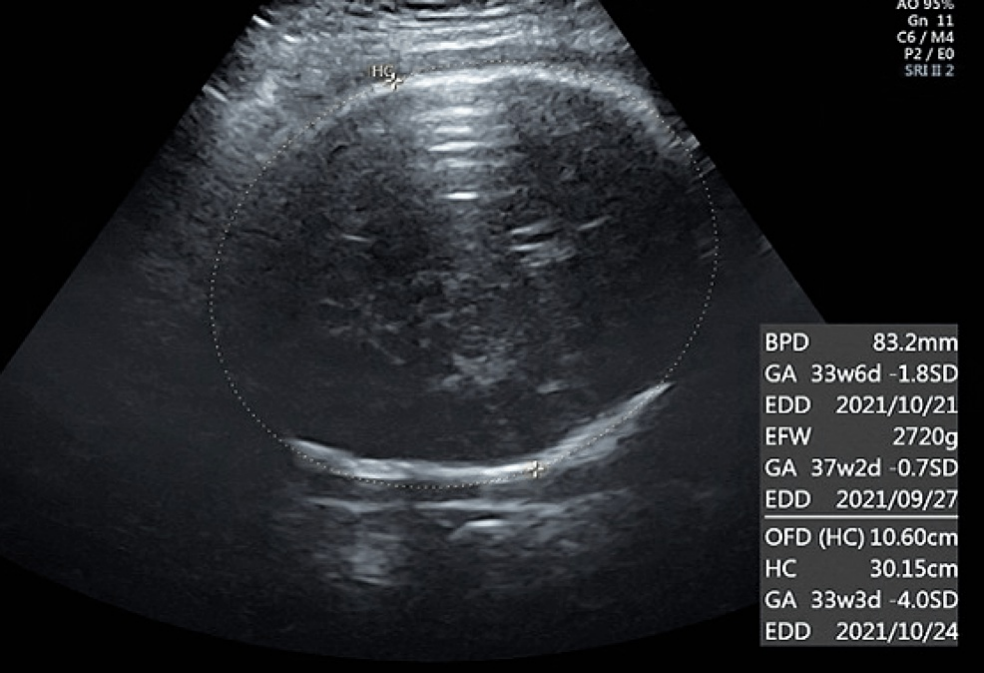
This is an ultrasound obtained at our clinic during the 40th week and 2nd day of pregnancy.
